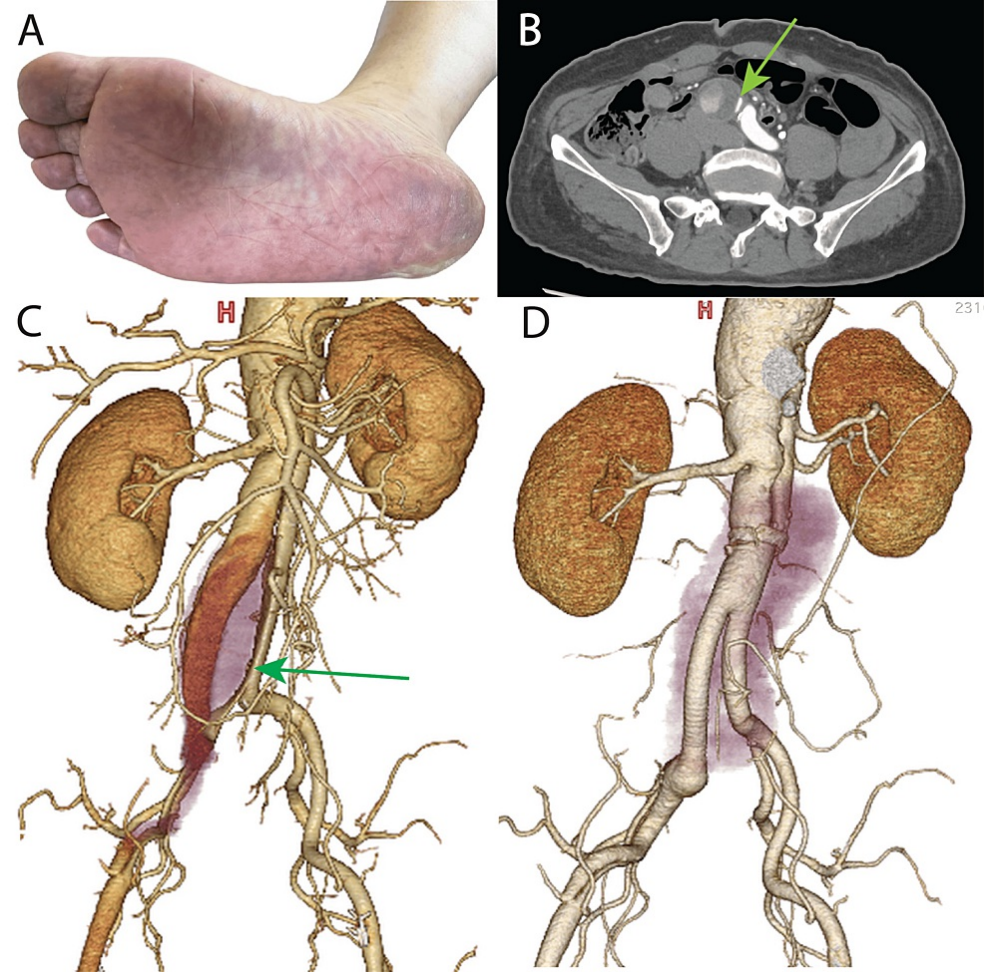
Preoperative findings (A) Discoloration of the sole of the foot was noted when symptoms of ischemia of the right lower extremity were present. The purple discoloration indicated ischemia. (B) Contrast-enhanced computed tomography at the appearance of symptoms of ischemia in the right lower extremity: the true lumen of the right common iliac artery was strongly compressed by the false lumen (arrow). (C) Contrast-enhanced computed tomography volume rendering before abdominal aortic artificial vascular replacement surgery: the abdominal aorta was dissected and extended to the right common iliac artery (arrow). The abdominal aorta itself had a diameter of 50 mm, which was an indication for surgery. (D) Contrast-enhanced computed tomography volume rendering after abdominal aortic artery replacement: the abdominal aorta was replaced with an artificial vessel.
